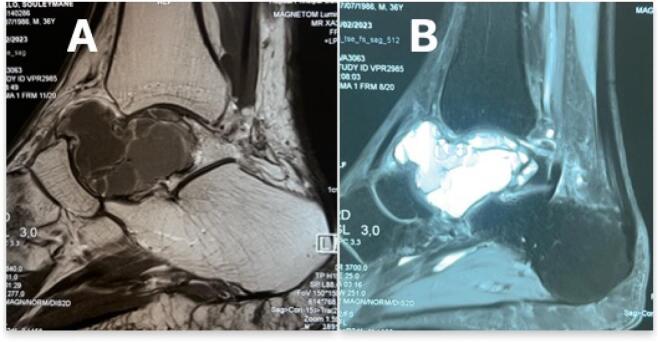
MRI of the left ankle. (A) Sagittal T1 MRI with contrast and fat suppression showing enhancement of the lesion with cortical.
